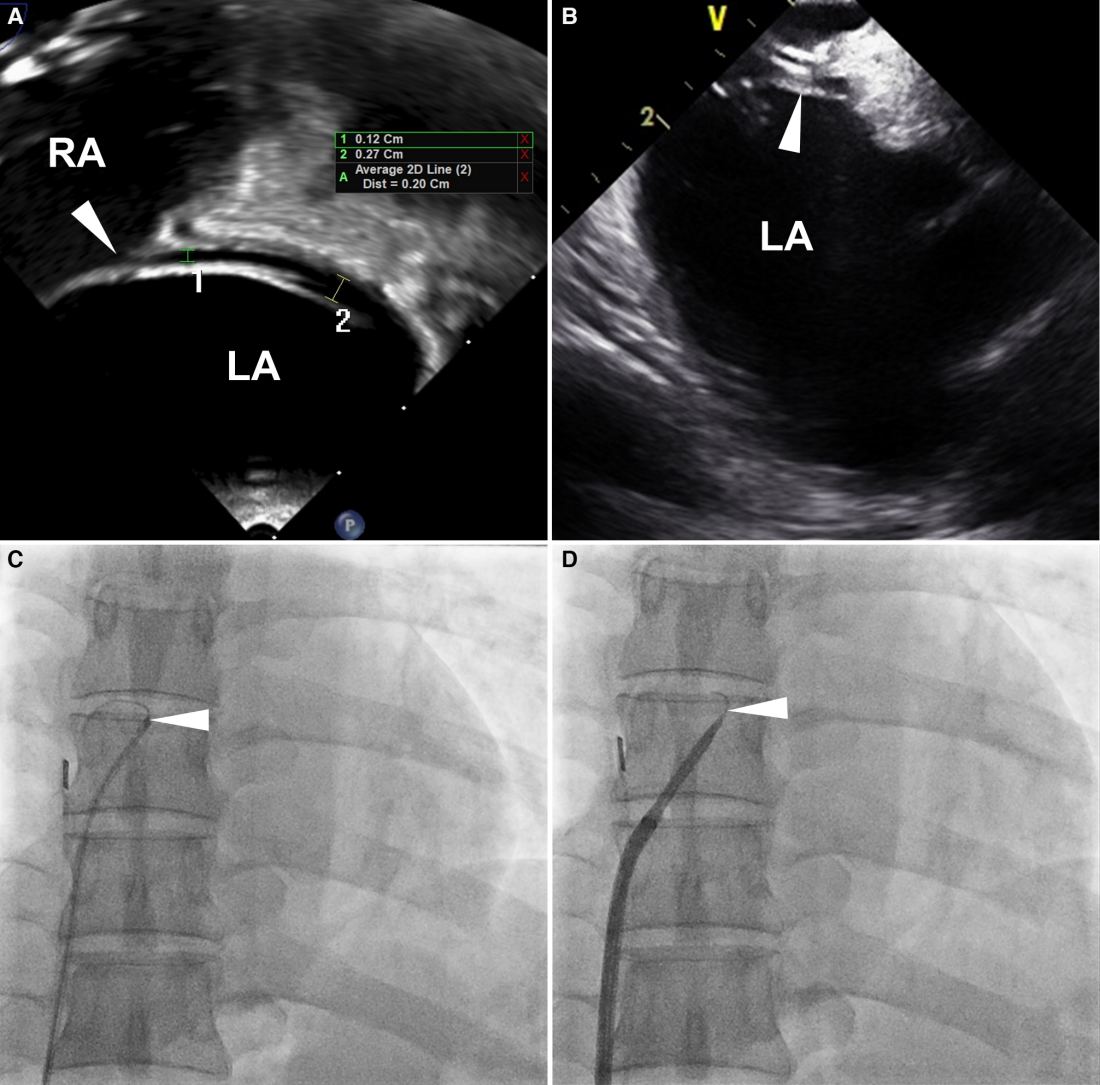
Definition of uncrossable PFO. Images from a 53-year-old man with an uncrossable PFO. (A) TEE 90-degree bicaval view shows a long-tunnel PFO. The probe was additionally rotated to optimize the visibility of the PFO tunnel in the bicaval view. (B) ICE imaging for PFO closure guidance. (C) 6 Fr MP catheter and 0.035-inch guidewire failed to pass through PFO. (D) SL1TM sheath replacement didn't resolve the issue. White arrowheads indicate PFO. ICE, intracardiac echocardiography; LA, left atrium; MP, multipurpose; PFO, patent foramen ovale; RA, right atrium; TEE, transesophageal echocardiography.
